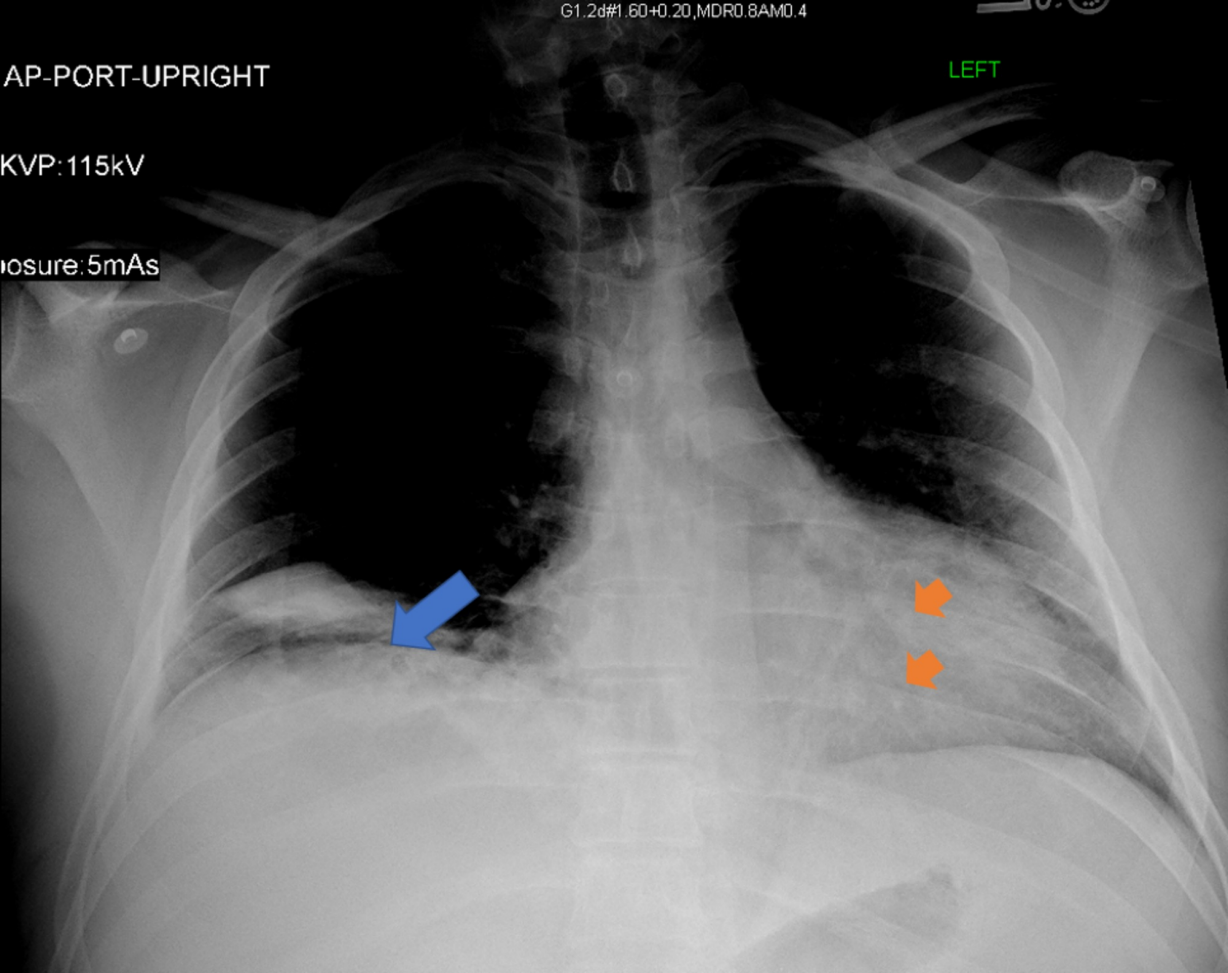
Chest X-ray Blue arrow pointing the pneumonic consolidation and orange arrows pointing some atelectasis
